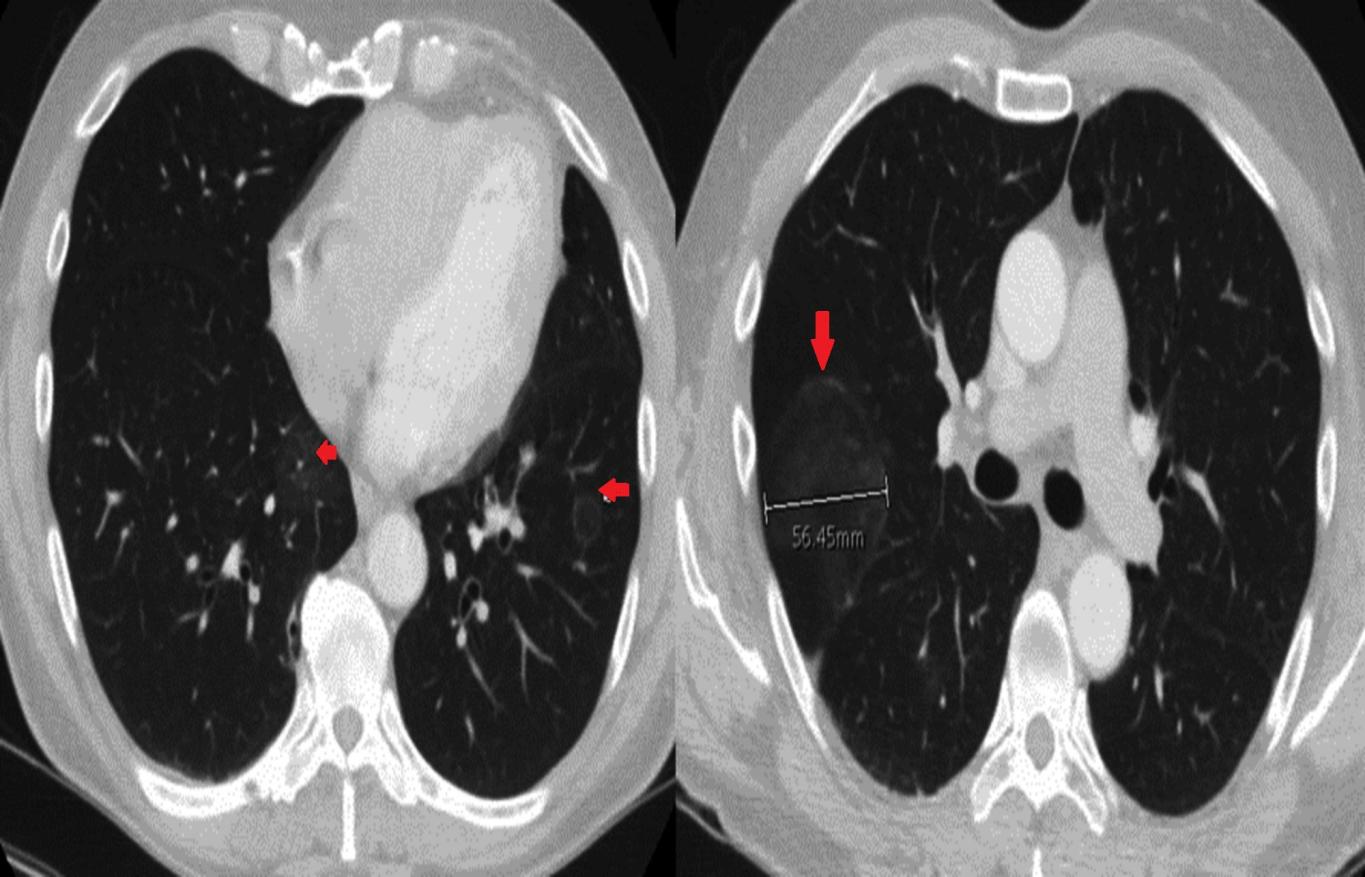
After three months: chest CT showing bilateral ground-glass opacities involving all lung lobes; the largest measured 5.7 cm in diameter CT - computed tomography
